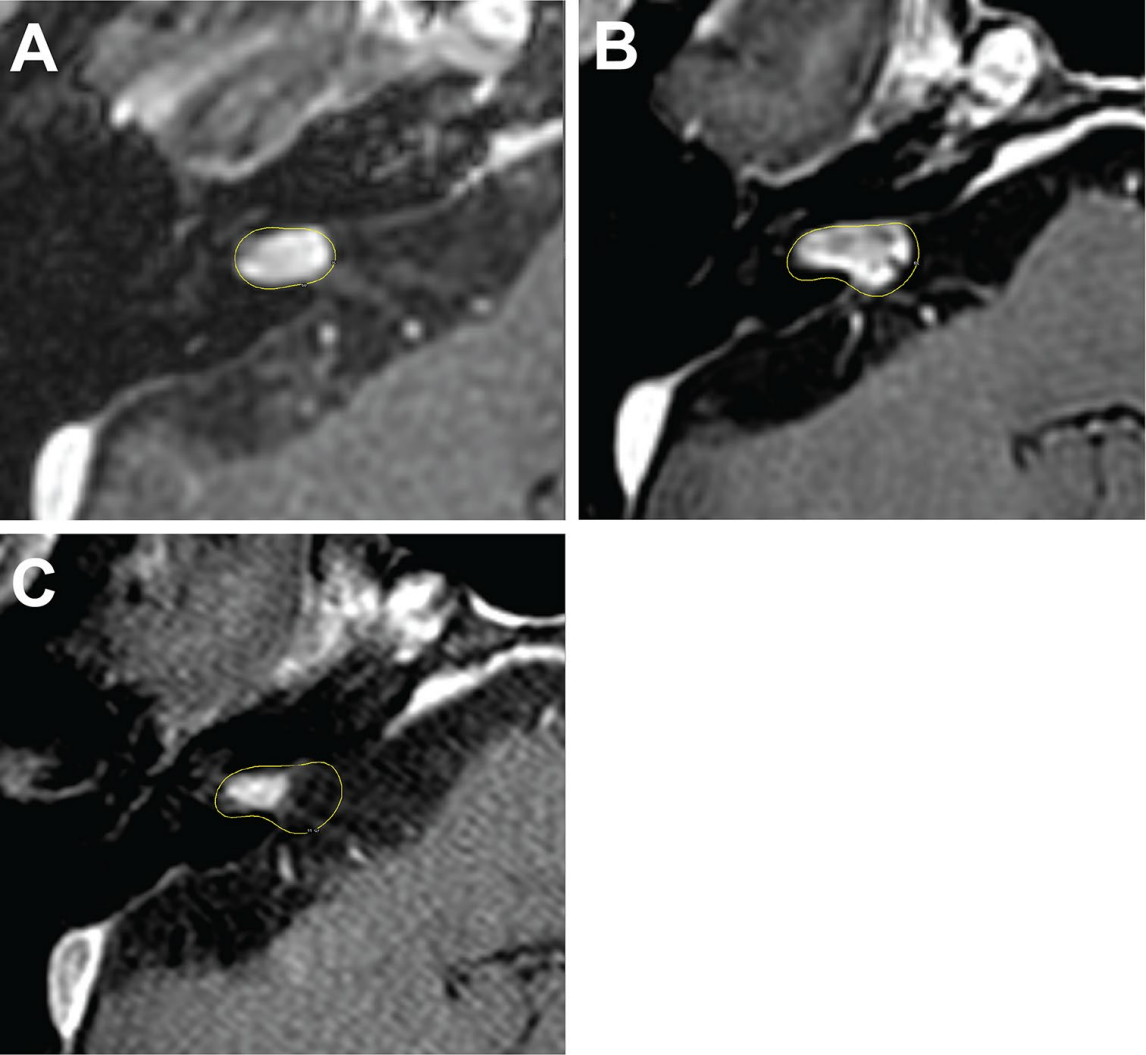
A 67-year-old male presenting with tinnitus and serviceable hearing underwent primary GKRS for a right sided vestibular schwannoma, sized 0.19 cc, as depicted in the axial and sagittal MRI sequence (Panel A). The tumor was initially targeted with a margin dose of 12 Gy at 50% isodose. The patient was serially monitored until developed persist tumor progression (0.42 cc) at 53 months after initial GKRS (Panel B). At this time, the patient’s tinnitus and serviceable hearing remained stable. The tumor in Panel B was managed with a tumor margin dose of 11 Gy at 55% isodose and a mean cochlear dose of 4 Gy. At last follow-up (Panel C), 47 months after the repeat GKRS, the patient’s vestibular schwannoma size had regressed (0.15 cc), and all clinical symptoms, including serviceable hearing, remained stable
